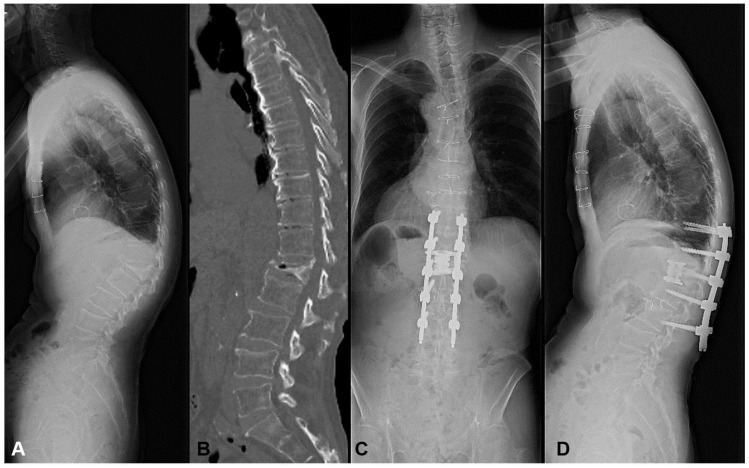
A 77-year-old male suffering from L1 vertebral collapse. The MIS L1 corpectomy, artificial body replacement, and L2/3 OLIF were performed followed by single-position percutaneous screw fixation. However, the distal screw pulled out at 1 month postoperatively. (A) Preoperative lateral X-ray, (B) Preoperative CT, (C) Standing AP X-ray at one month postoperatively, (D) Standing lateral X-ray at one month postoperatively.
